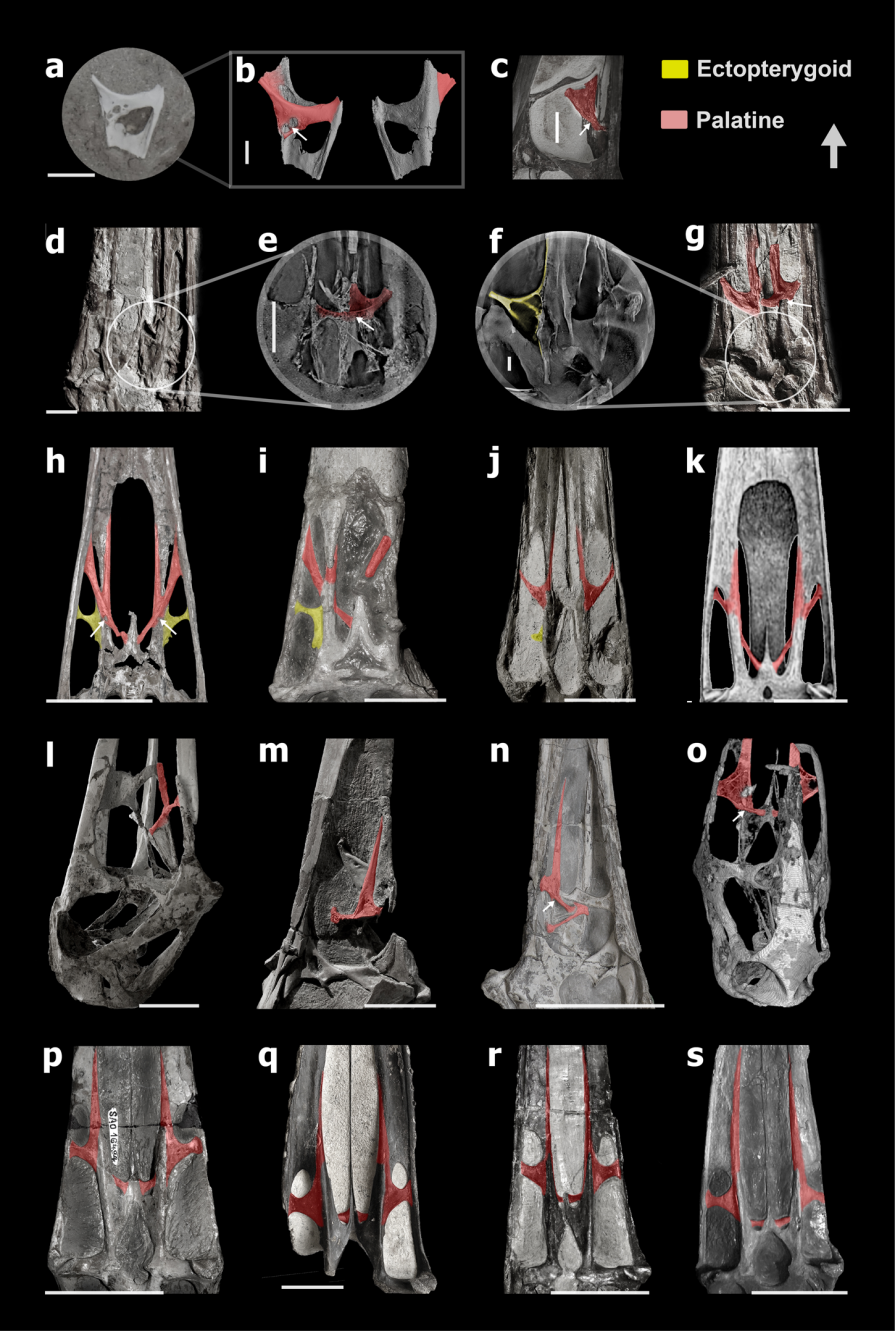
The similar structure on the palatal region in pterodactyloids. a A piece of skull of *Hamipterus tianshanensis* (left side) IVPP V 18943.3. b CT-rendered bone corresponding to the (a) in dorsal and ventral view. c A close-up shot of the palatine of *Ludodactylus* SMNK PAL 3828 in dorsal view. d the posterior part of the palate of *Hongshanopterus lacustris* IVPP V 14852 in ventral view, modified from ref. ^35^. e CL-scan results corresponding to the circle area in (d). f CL-scan results corresponding to the circle area in (g). g The posterior part of palate of *Kunpengopterus sinensis* IVPP V 23674 in dorsal view, modified from ref. ^41^. h The posterior part of the palate of *Caupedactylus ybaka* MN 4726-V in dorsal view, modified from ref. ^38^. i The posterior part of the palate of *Tupuxuara leonardii* IMCF 1052. j The posterior part of the palate of *Gnathosaurus subulatus* SOS 4580 (cast) in ventral view. k The posterior part of the palate of *Pteranodon*, modified from ref. ^64^. l–r Seven skulls of *Anhanguera* focus on the palate region, and they are RGM 401880 (lateral-dorsal view on the right side, photographed by Dr. T. Rodrigues), AMNH 22555 (lateral view on the right side), SNSB-BSPG 1982 I 89 (lateral view on the left side), AMNH 25555 (dorsal-lateral view on left side, CT-rendered model modified from ref. ^33^, SAO 16494 (ventral view, photographed by Dr. T. Rodrigues), SNSB-BSPG 1982 I 89 (ventral view, photographed by Dr. T. Rodrigues), MN 4805-V (ventral view), respectively. s The posterior part of palate of *Tropeognathus mesembrinus* BSP 1987 I 46 (cast) in ventral view. Scale bar: a, d, e, g = 10 mm; b = 4.2 mm; j = 20 mm; f = 1 mm; h, i, k, l, m, p, q, r = 50 mm; n = 100 mm; s = 85 mm.
